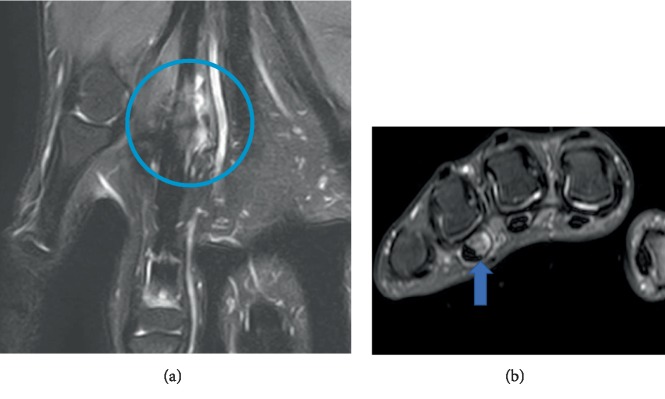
(a) MRI T2 sequence: coronal view of the hand. (b) MRI T2 sequence: axial view of the hand.
